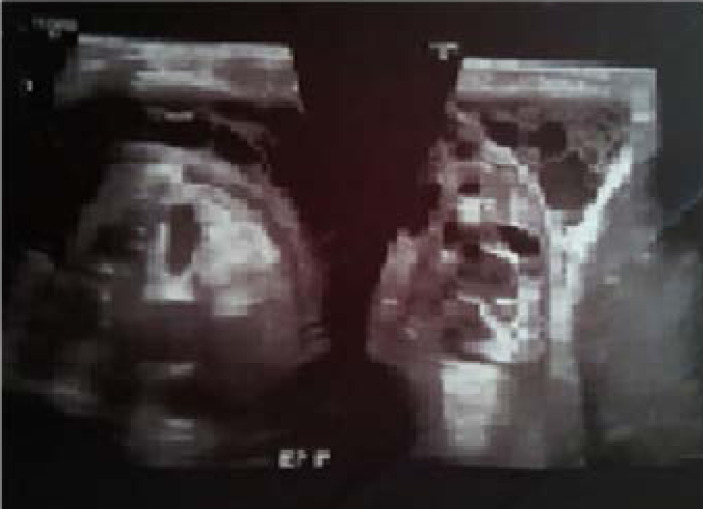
Chest ultrasonography showing multiple cysts occupying almost all of the left hemithorax associated with pleurisy
